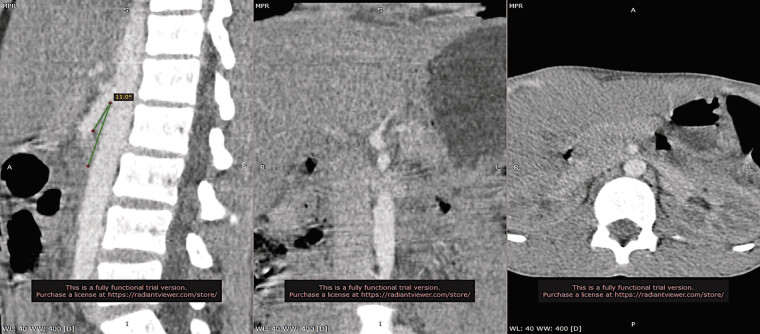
Contrast-enhanced computed tomography (CT) images showing anterior nutcracker syndrome. The sagittal view demonstrates a narrowed aortomesenteric angle, and the axial view reveals the classic “beak sign” due to compression of the left renal vein (LRV).
